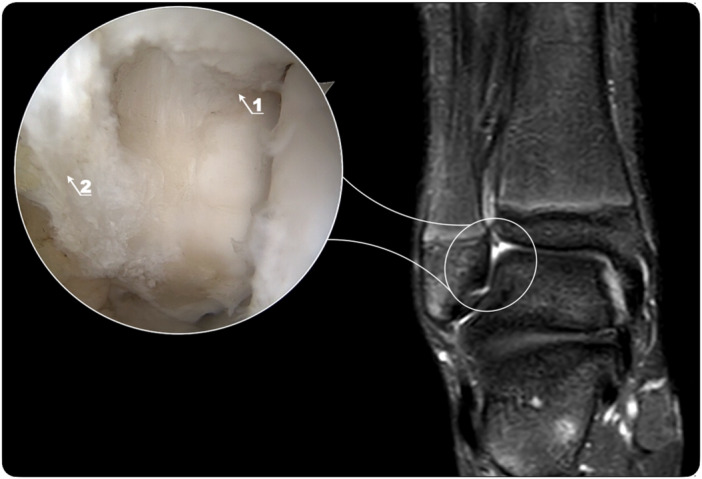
Preoperative MRI (right) and corresponding arthroscopic view of the lateral gutter (left) showing the fibular physis (1) at the same level as the joint line and located behind the anterior inferior tibiofibular ligament (2). MRI, magnetic resonance imaging.
